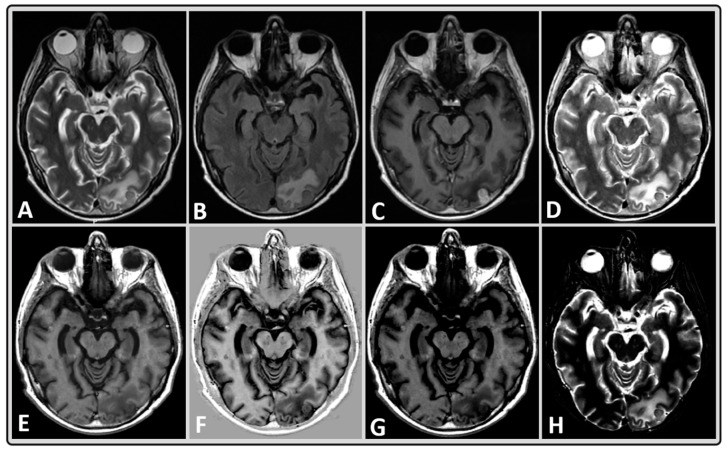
Representative MRI data of a 40-year-old male BM patient treated with whole-brain RT using standard and synthetically-generated (MAGiC) images. (A–C) T2w, T2w fluid attenuated inversion recovery (FLAIR) and T1w post-contrast images, respectively, from standard clinical imaging. (D–H) T2w, T1w, phase-sensitive inversion recovery (PSIR), T1w FLAIR, and short tau inversion recovery (STIR) images, respectively, from MAGiC.
